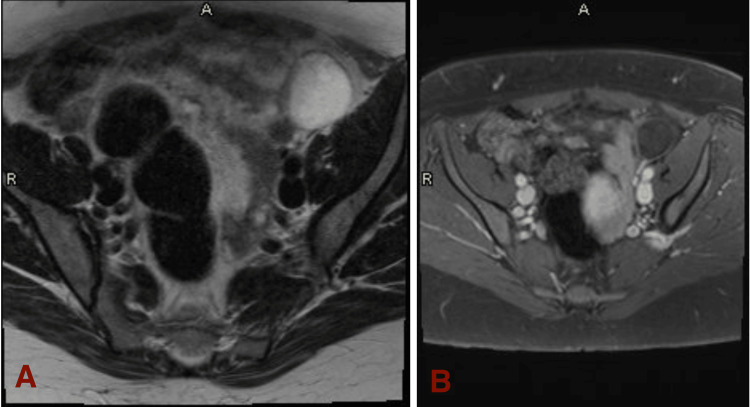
T2-weighted MRI (A) is shown on the left, expressing a simple cyst in the left ovary, measuring about 4 cm in length. The cyst is located in the top right section of the image, white in color. In contrast, the T1-weighted MRI (B) is shown on the right, expressing the same findings but is dark in color. Both MRI images are for Patient B.
